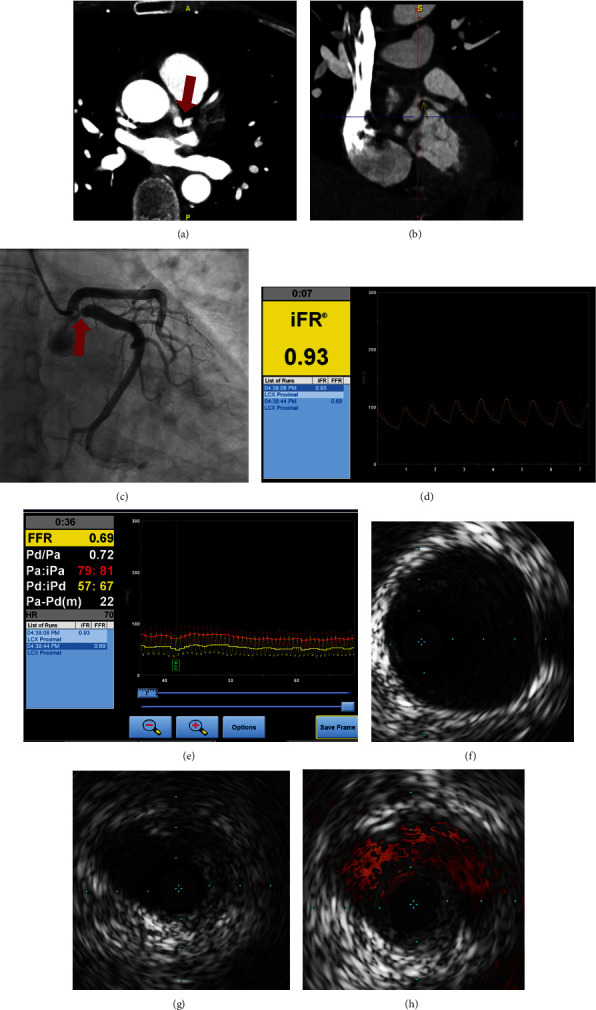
(a and b) Coronary CT angiography demonstrating proximal left circumflex artery blockage. (c) Coronary angiography showing 70% stenosis of the left circumflex artery. (d and e) Discrepant iFR and FFR results. (f) Intravascular ultrasound showing normal reference segment distal to the proximal lesion. (g) Eccentric narrow orifice without atherosclerotic plaque with a minimal lumen area of 6.7 mm^2^. (h) Color-flow image of eccentric narrow orifice without evidence of atherosclerotic plaque.
